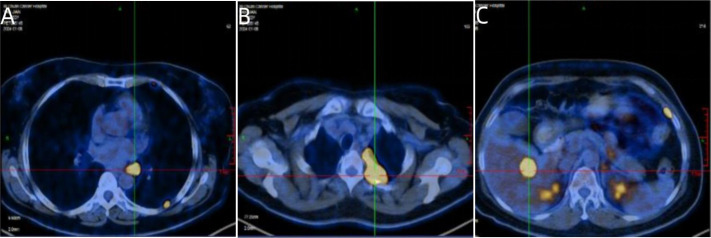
Representative PET-CT images at initial diagnosis. (A) Axial PET-CT image showing enlarged left hilar lymph nodes. (B) Axial PET-CT image demonstrating multiple pleural nodules along the left pleura. (C) Axial PET-CT image revealing a hepatic metastatic lesion in the right lobe of the liver.
